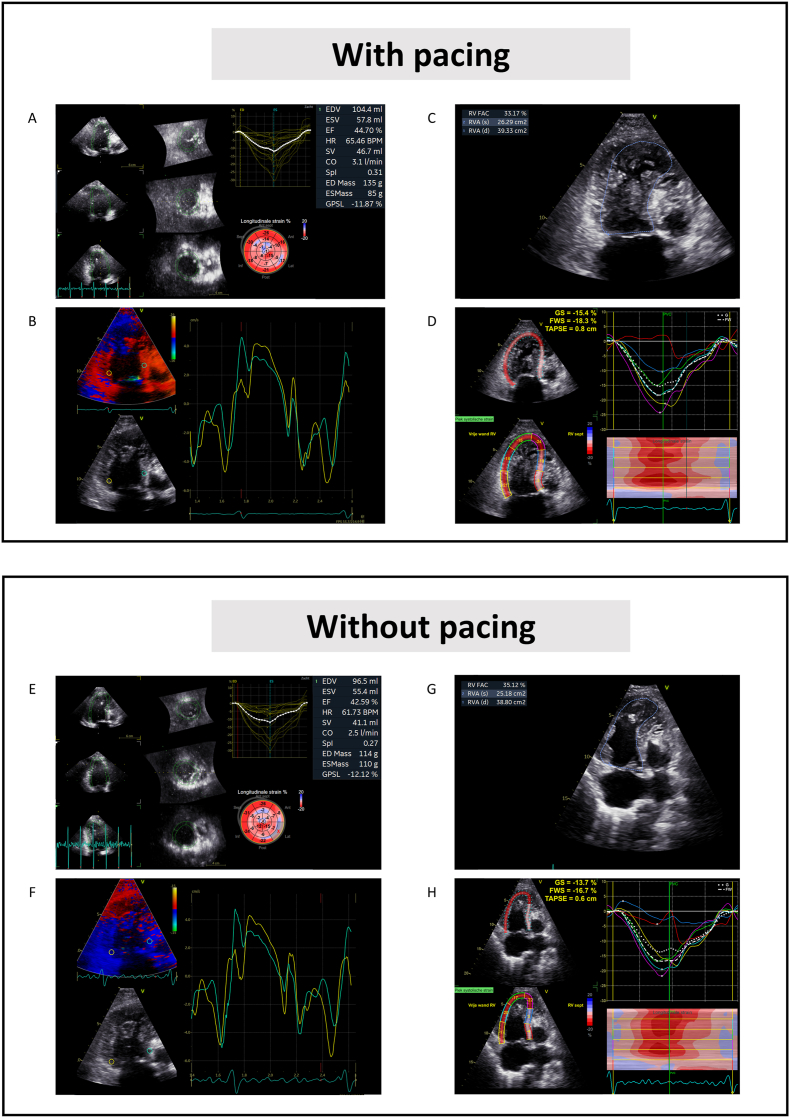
Outpatient clinic echocardiography performed at 2.5 years of follow-up. sRV systolic function remained unchanged compared to preoperative status (C and D with pacing and G and H without pacing). Although 3D regional volumetric curves showed only a slight delay with pacing compared to without pacing (A vs. E), no measurable septal-to-lateral delay was observed with tissue doppler imaging with and without pacing (B vs. F).
